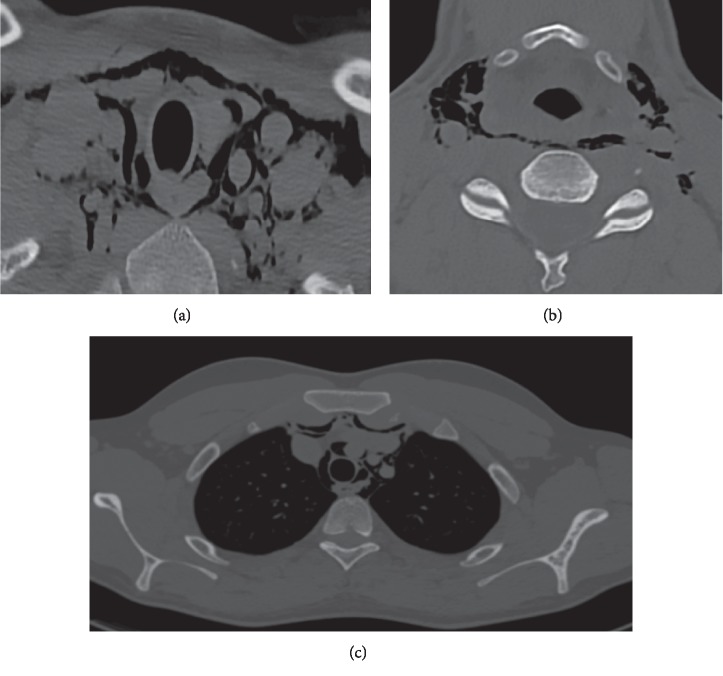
Contrast enhanced CT scan axial images for patients with spontaneous subcutaneous emphysema of the neck and anterior medistinum. (a) Emphysema occurring in neck spaces: parapharyngeal, carotid, and pretracheal spaces. (b) Emphysema in retropharyngeal space with parapharyngeal extension. (c) Emphysema also extending to mediastinal space and some air pockets are seen retrosternal.
